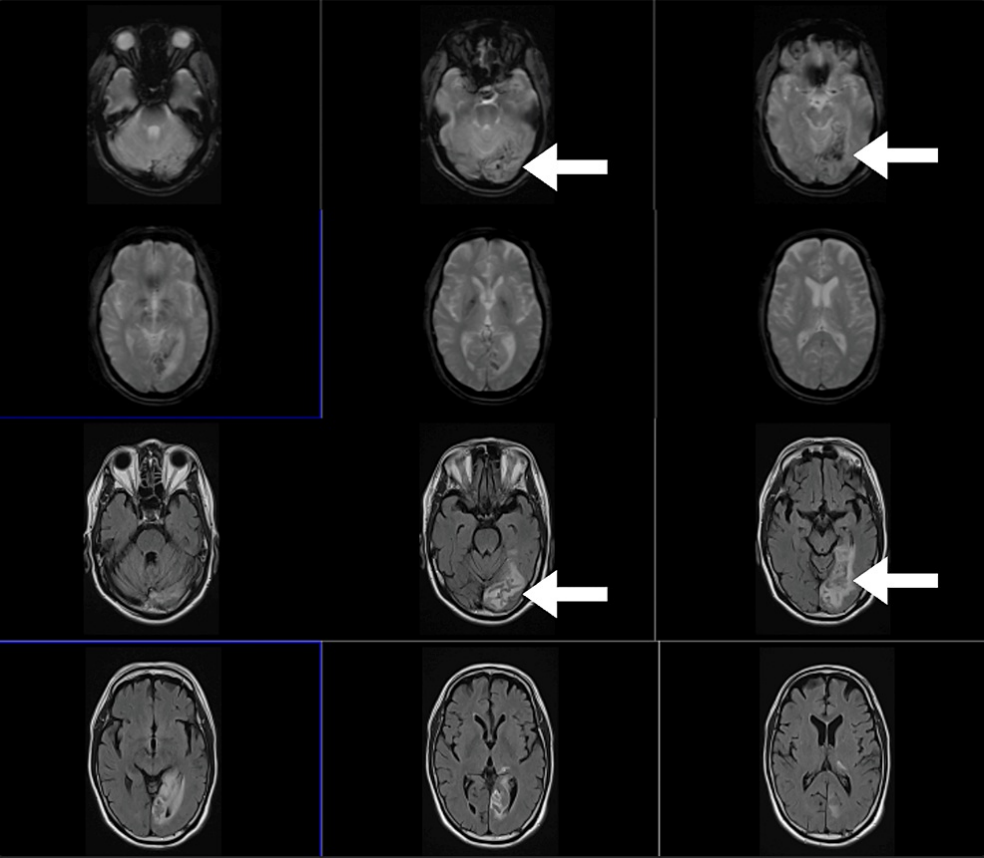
Cranial MRI (GRE – top; T2 FLAIR – bottom) showing a subacute hemorrhagic infarct involving the left posteromedial temporal and left occipital lobes and to a lesser extent the left posterior thalamus GRE: gradient recalled echo; FLAIR: fluid-attenuated inversion recovery
